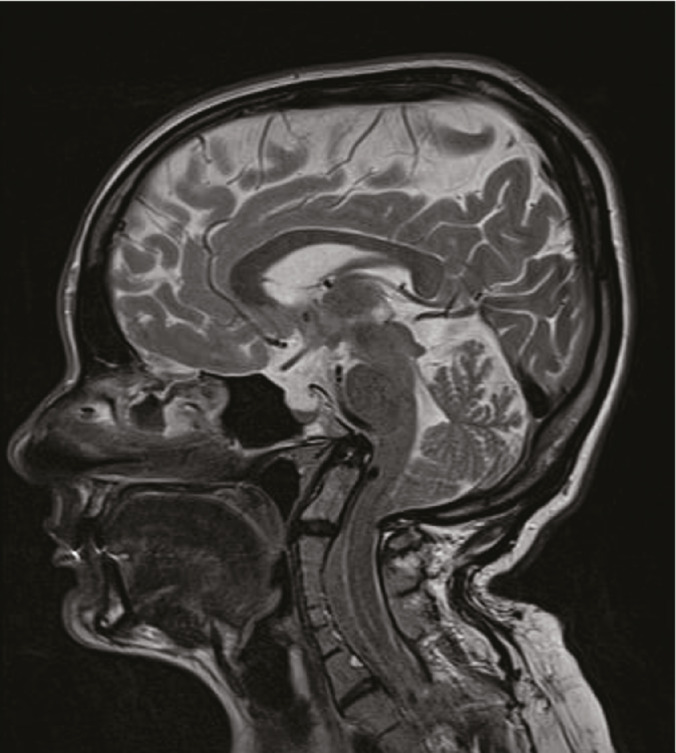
Pre-pregnancy magnetic resonance imaging showing fusion of vertebrae C3 to Th1.
